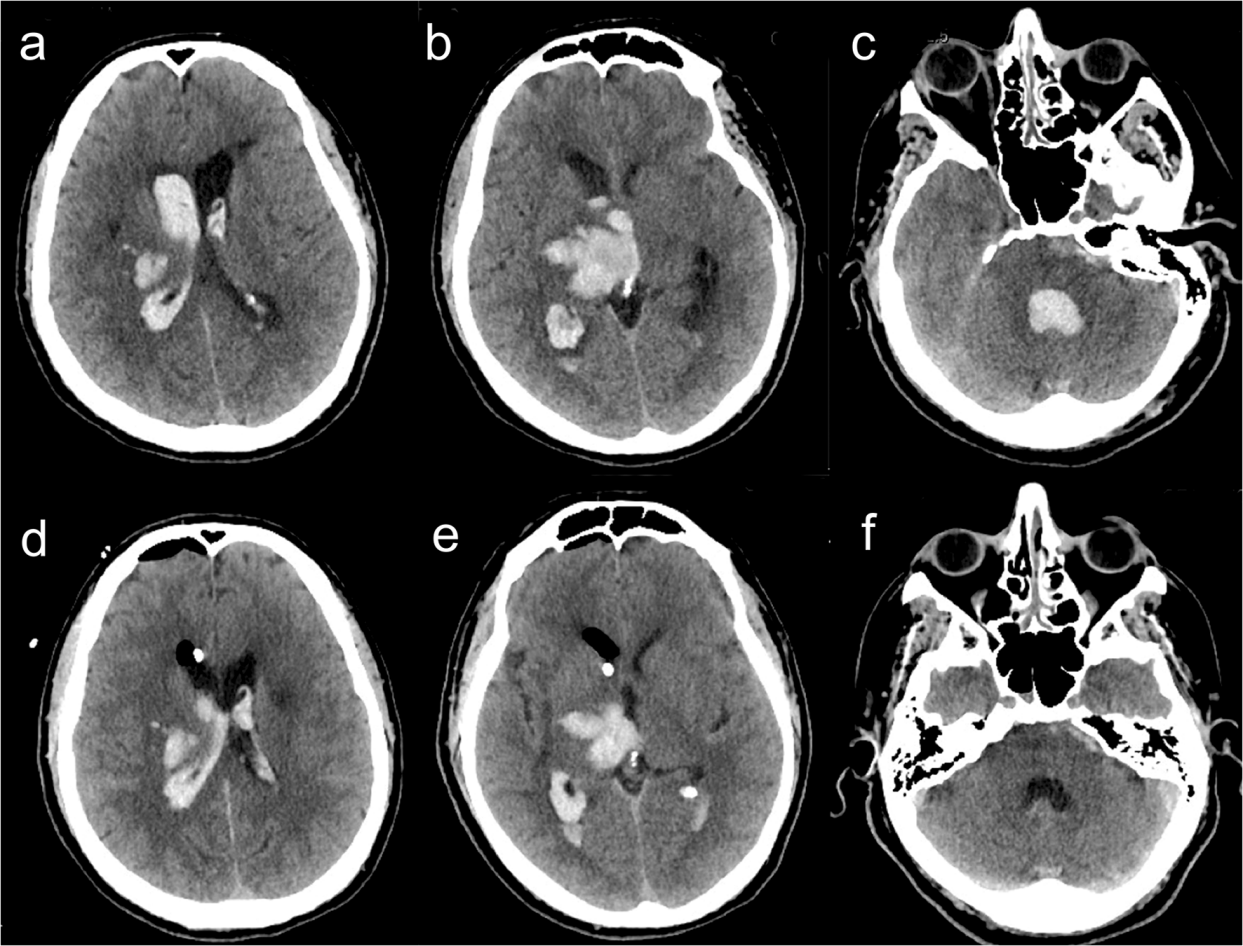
a, b, c Preop CT scan showing a thalamic hemorrhage with intraventricular extension. d, e, f Immediate postop CT scan after endoscopic IVH aspiration
